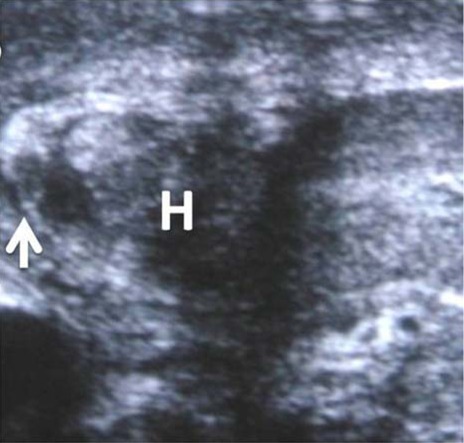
Transverse ultrasound image shows intracavernosal haematoma (H) and the site of ruptured tunica (marked by an arrow).
